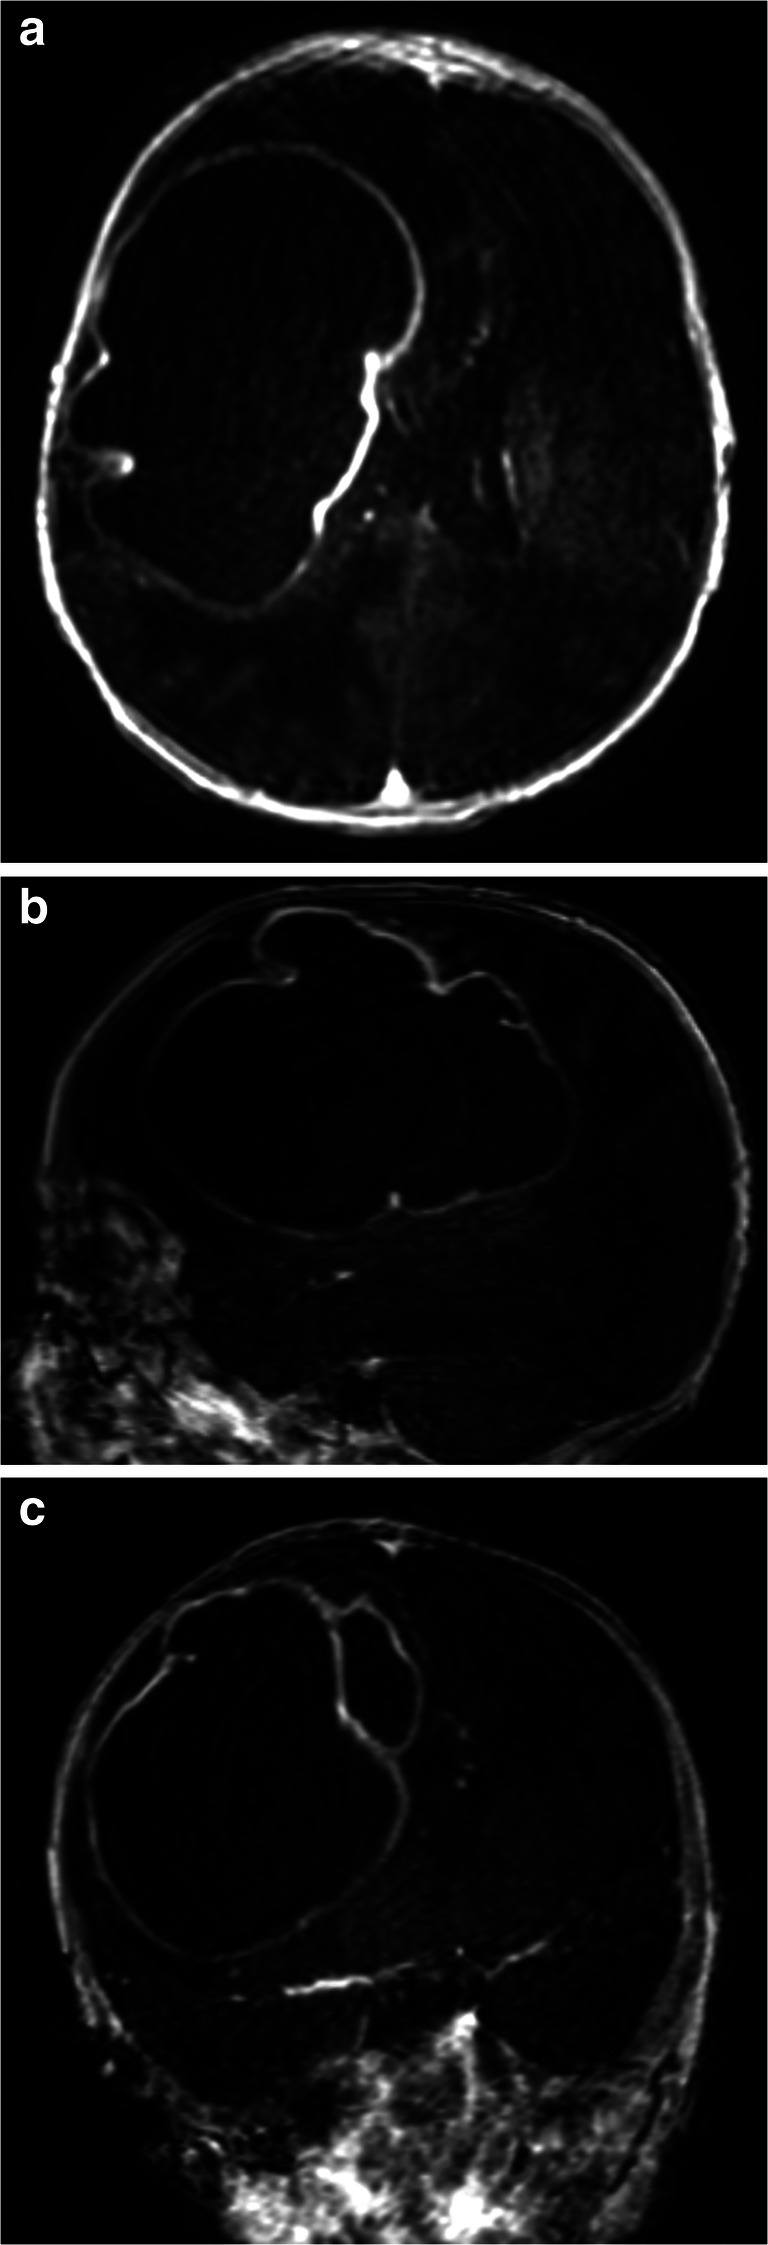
T1-weighted MRI with contrast showing a ring enhanced lesion in the right hemisphere with surrounding edema and midline shift. Mass dimensions: 78×51×58mm with an estimated volume of 120 ml. a Axial view. b Sagittal view. c Coronal view
